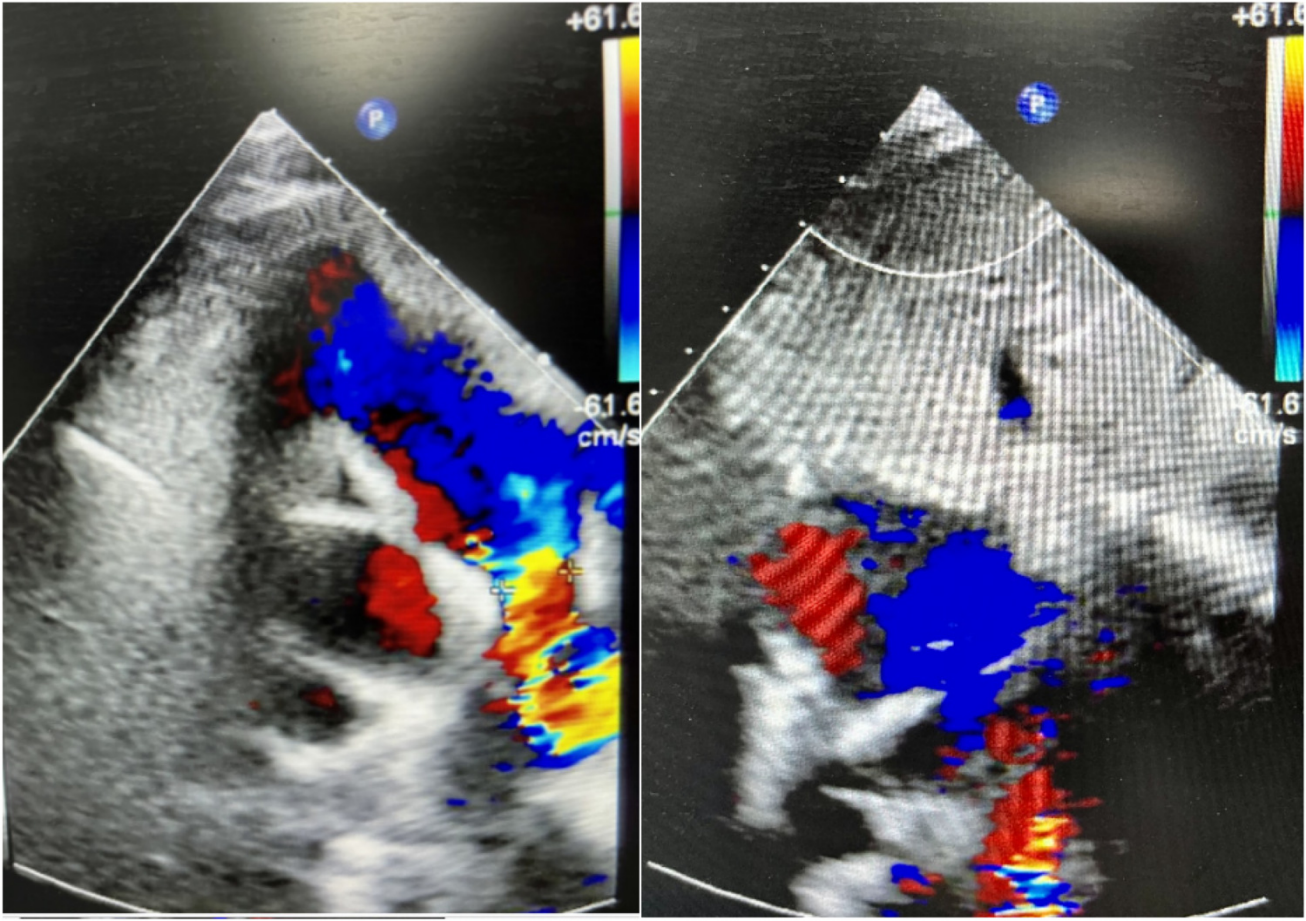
Cardiac ultrasound of case 1 at guangdong provincial people's hospital (January 11, 2024).
